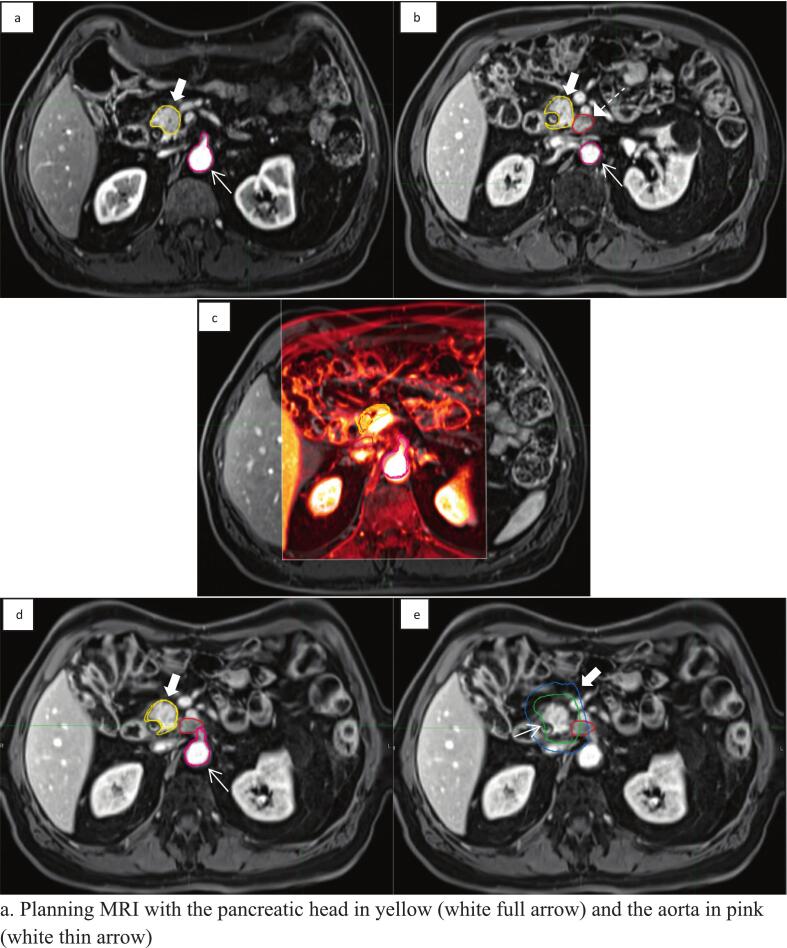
Deformable registration process. a. Planning MRI with the pancreatic head in yellow (white full arrow) and the aorta in pink (white thin arrow). b. Recurrence MRI with the pancreatic head in yellow (white full arrow), the aorta in pink (white thin arrow) and the loco-regional recurrence in red (white dashed arrow). c. Reg Refine Tool with a box centred on a Region of Interest, locking points of interest. d. Final deformed MRI, with both contours appearing on the image. e. Final deformed MRI, with the projected ID35 in green (thin arrow), the projected ID25 (full arrow) in blue and loco-regional recurrence in red. (For interpretation of the references to colour in this figure legend, the reader is referred to the web version of this article.)
